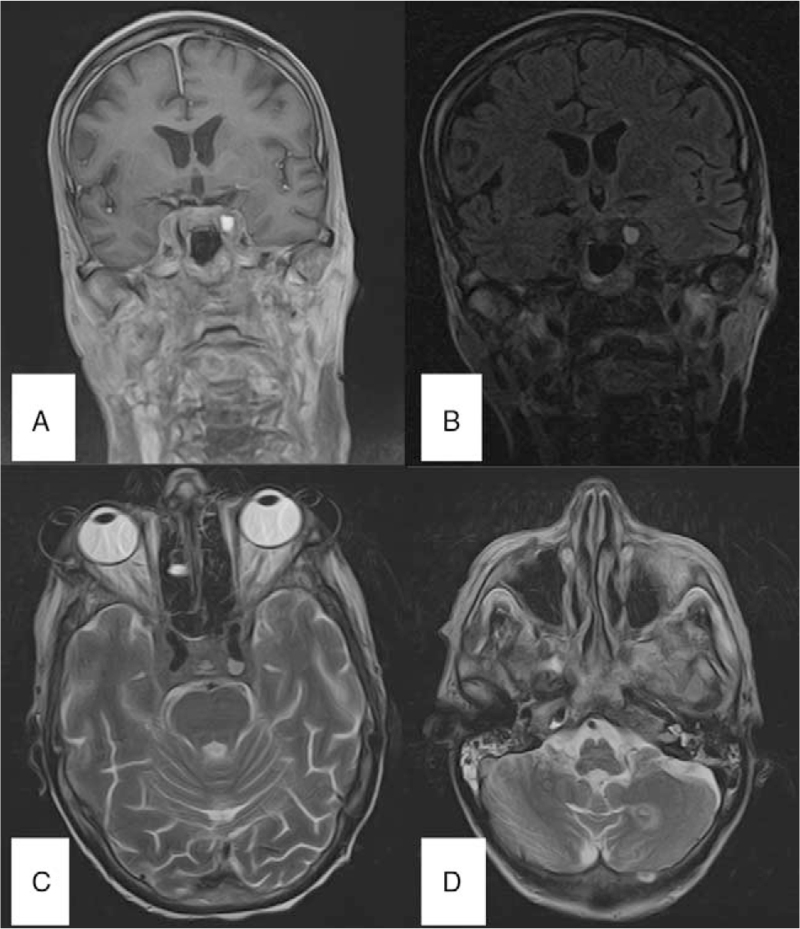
Cerebral magnetic resonance imaging scan – T1-weighted (A), FLAIR (B) coronal sections and T2-weighted (C, D) cross-sections showing 2 non-contrast enhancing T2-weighted/FLAIR hyperintense lesions in the left cavernous sinus and the left cerebellar hemisphere.
